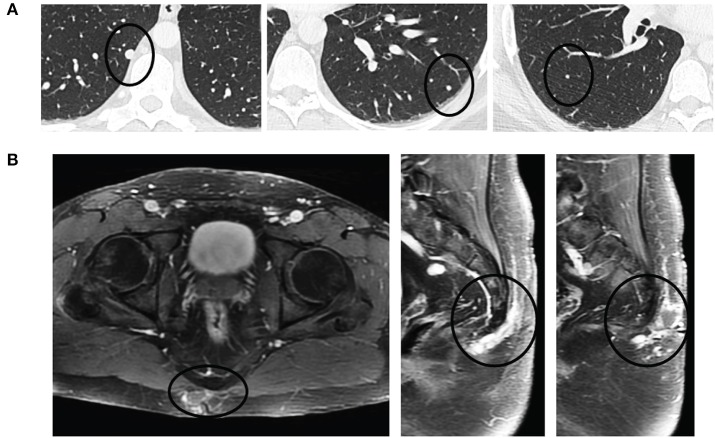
Pulmonary oligometastases upon detection of recurrent sacrococcygeal mass. (A) Axial views of the lungs revealing a small, discrete homogeneously hyperdense nodule (black circle) in the right lower lobe (left) that had doubled in size since initial resection (8 mm from 4 mm) and enlarging left lower lobe (middle) and right lower lobe (right) metastases. (B) Recurrent post-sacral mass on axial (left) and sagittal (middle, right) fat-saturated contrast-enhanced T1-weighted sequences is heterogeneously enhancing and multilobulated in appearance as it extends through the soft tissues without invasion into the coccyx.
